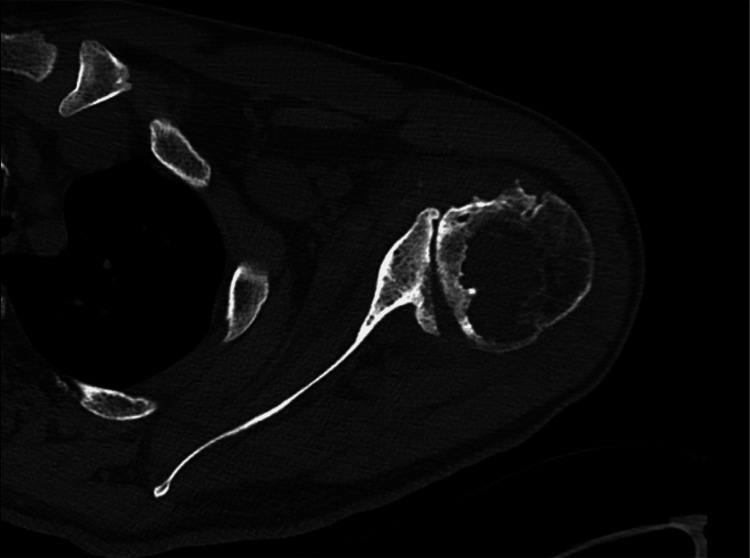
Preoperative CT of the left shoulder CT: computed tomography The image depicts an axial view of the right shoulder
